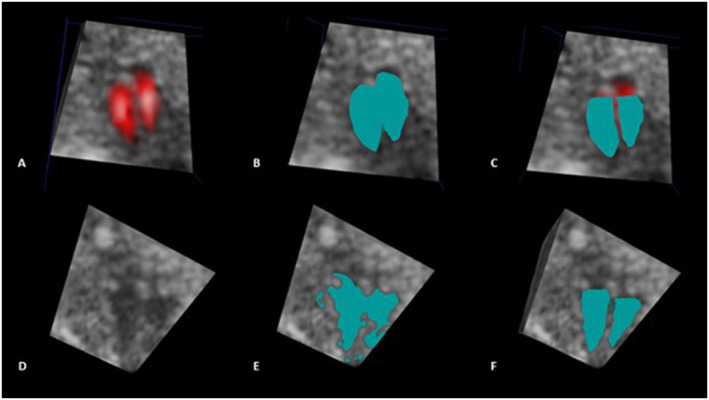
Infographic (panel A to F) showing how Virtual Reality (VR) was applied to perform fetal cardiac ventricle volume (FCVV) measurements. Panel (A–C) FCVV measurements performed in color Doppler mode (VR‐CD). (A) A Spatio‐Temporal Image Correlation (STIC) ultrasound (US) dataset displayed in color Doppler (CD) mode (based on atrioventricular blood flow). (B) FCVV measurement performed using VR in color Doppler (VR‐CD) mode. The colored (cyan) regions indicate the segmented region of the cardiac ventricle after using an automated segmentation algorithm based on a pre‐set number of voxel (red or blue‐) value thresholds. (C) The colored (cyan) regions indicate the segmented region of the cardiac ventricle after manually removing incorrect (un)segmented voxels from the segmented region in “B”. Panel (D–F) FCVV measurements performed in gray‐scale mode (VR‐GS). (D) A STIC US dataset displayed in gray‐scale (GS) mode. (E) FCVV measurements performed using VR in gray‐scale (VR‐GS) mode. The colored (cyan) regions indicate the segmented region of the cardiac ventricle after using an automated segmentation algorithm based on a pre‐set number of voxel (gray‐)value thresholds. (F) The colored (cyan) regions indicate the segmented region of the cardiac ventricle after manually adding or removing incorrect (un)segmented voxels from the segmented region in “E”.
